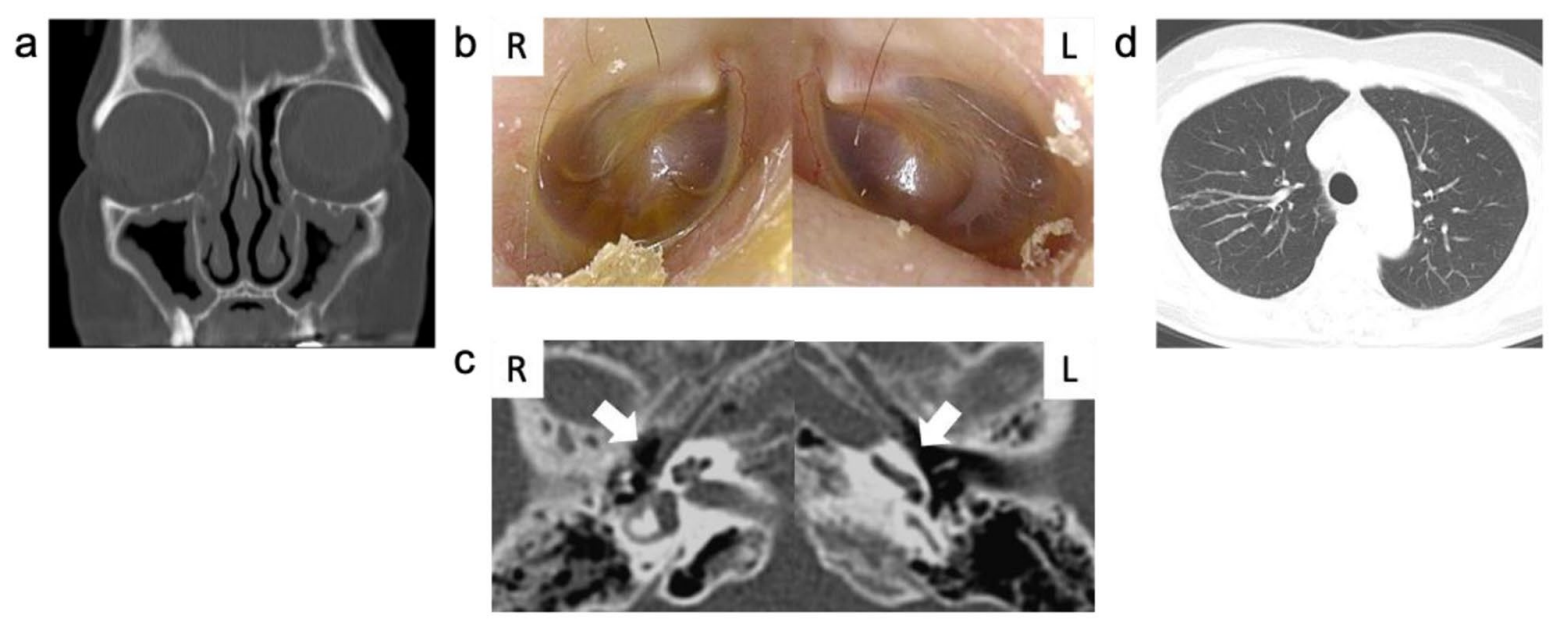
Computed tomography (CT) images of sinuses, temporal bone, and chest, with otoscopic findings before initiation of dupilumab Before dupilumab administration, eosinophilic chronic rhinosinusitis (ECRS) relapse was observed. Sinus CT showed bilateral soft tissue shadows predominantly in the right frontal sinus (a). Brown middle ear effusion was partially observed predominantly in the right ear (b). Temporal bone CT slightly showed soft tissue shadows in the right tympanic cavity. White arrows indicate the tympanic cavity (c). Chest CT scan did not show any consolidations (d)
